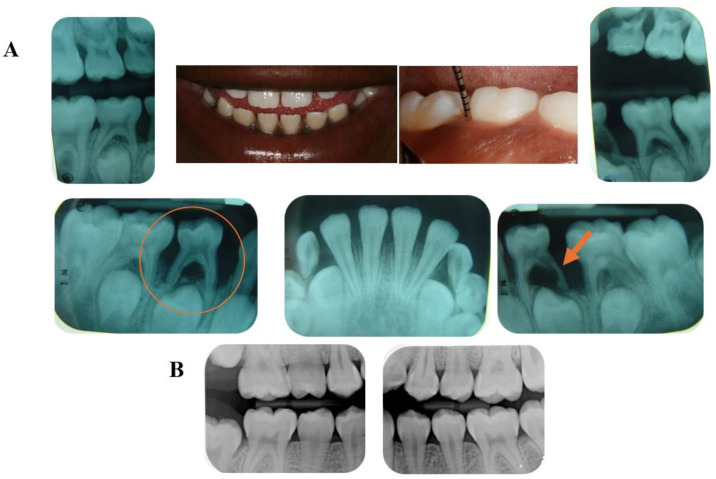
Clinical Case: 8-year-old African American female diagnosed with C-MIP in primary dentition. Patient smiles with low gingival margin inflammation; however, probing depth > 5 mm in the first molar. In the X-ray, severe bone loss in the lower first primary molars (orange arrow and circle) along with internal and external resorption of the lower left primary first molar in the primary dentition (orange arrow) (A). Permanent dentition in healthy conditions following therapy (SRP+ABX) in the primary dentition (B). (Source of the image [10]).
